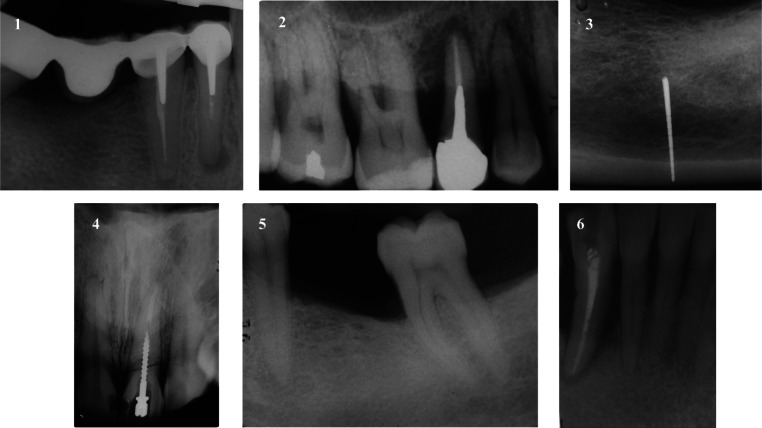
Periapical x-rays of the 6 patients prior to implant placement. In the first patient, teeth were extracted for endodontic treatment failure, they both presented periapical lesions. In the second patient, tooth 1.5 was extracted for endodontic failure. The third patient had his tooth (3.7) extracted for severe periodontitis. In the fourth patient, tooth 2.1 had a horizontal fracture and a crack over the root due to the endocanalar post displacement. Fifth patient had his tooth (3.6) extracted for periodontitis. The sixth patient lost his tooth (4.3) due to a severe endodontic-periodontal lesion; the tooth was necrotic and presented a visible periapical lesion
